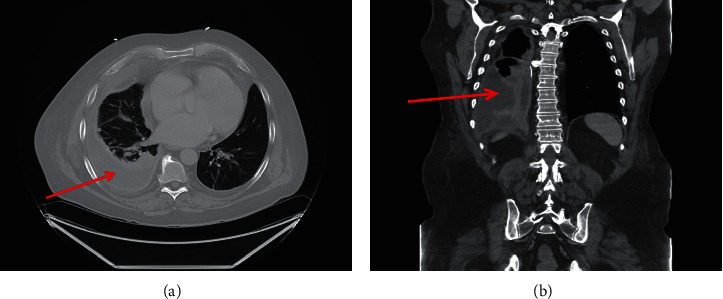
CT chest with contrast of the patient taken shortly after admission demonstrating pleural effusion in the (a) axial and (b) coronal views. The red arrow denotes pleural effusion.
